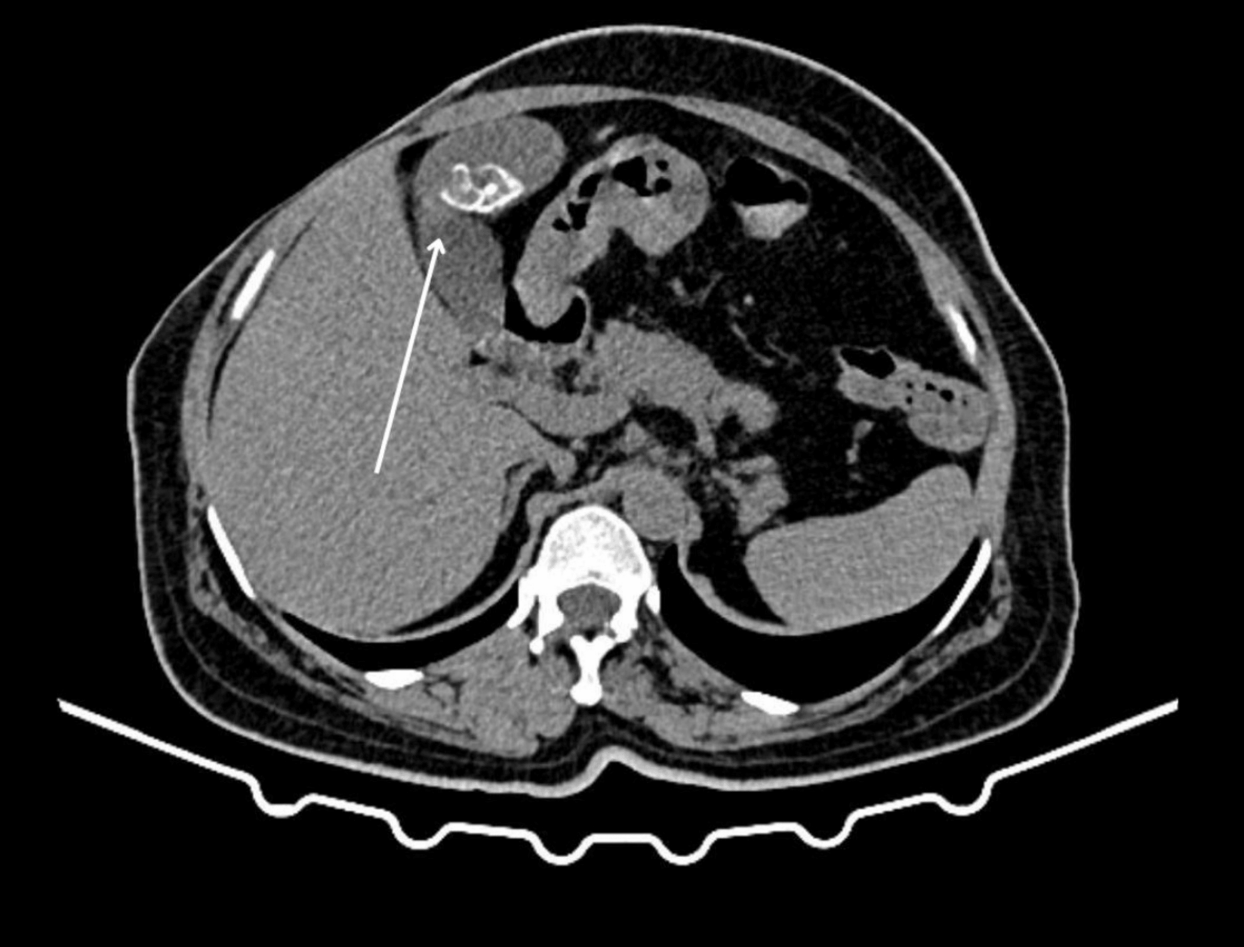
CT image showing the gallbladder with calculi (marked with a white arrow)
